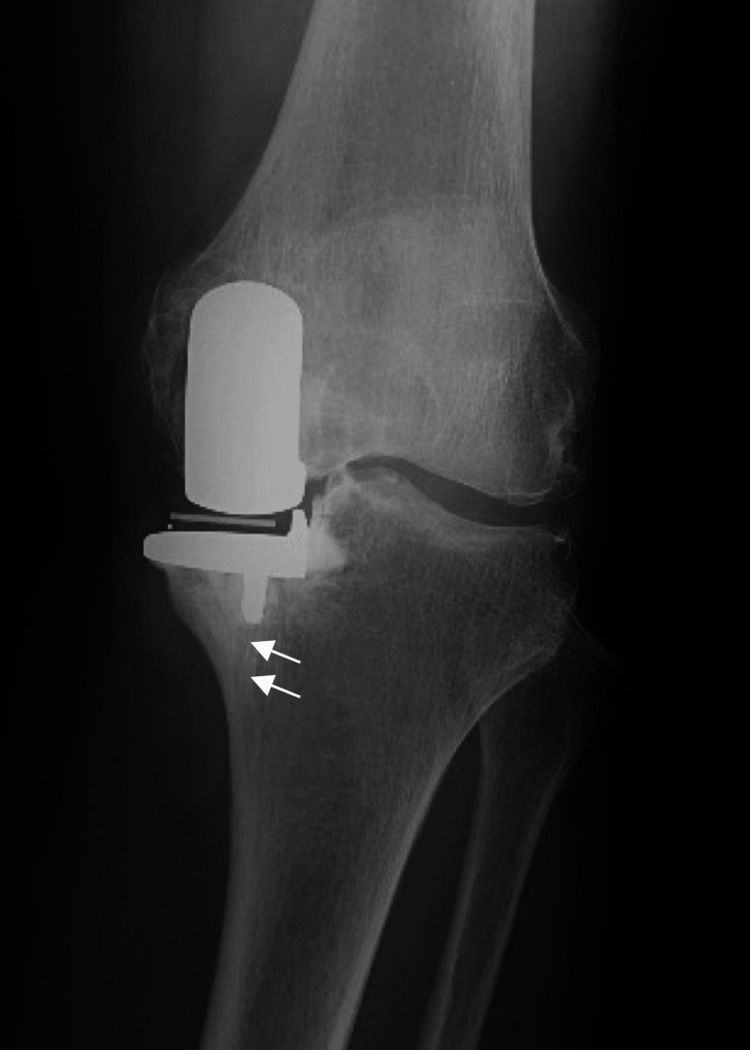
Four years postoperative radiography of the peri-implant fracture
